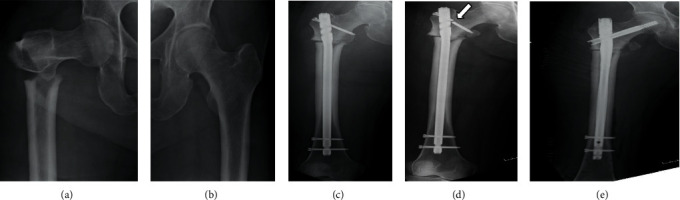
Radiographs of both femurs. The initial right femoral plain radiograph shows an atypical subtrochanteric fracture (a). On the contralateral side, no fracture is detected at the subtrochanteric site (b). The intramedullary nail is inserted during primary surgery with osteosynthesis (c). The intertrochanteric screw is broken (white arrow) (d), and the fracture site is more displaced than that shown in (c). Revision surgery is then performed. The intramedullary nail is reinserted, and the lag screw is inserted in the femoral neck and head (e).
